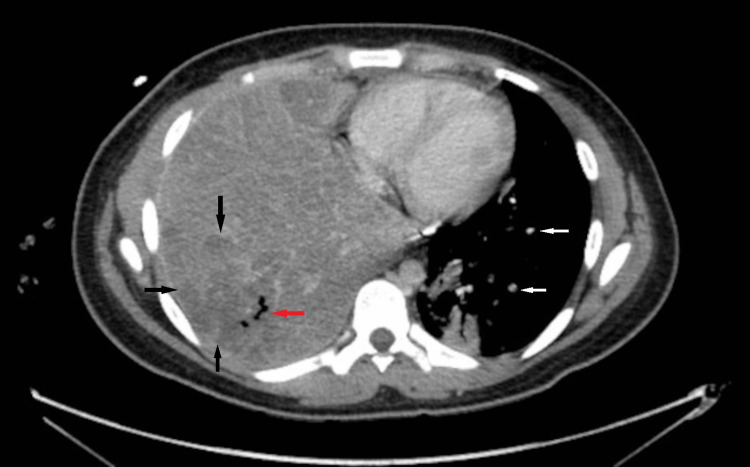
CT of the chest There was complete consolidation of the right middle and lower lobes with subtotal consolidation of the right upper lobe. Multifocal areas of diminished parenchymal enhancement (black arrows) and a few small areas of parenchymal cavitation (red arrow) in the right middle and lower lobes were observed. There were nodular opacities in the left lower lobe (white arrows).
